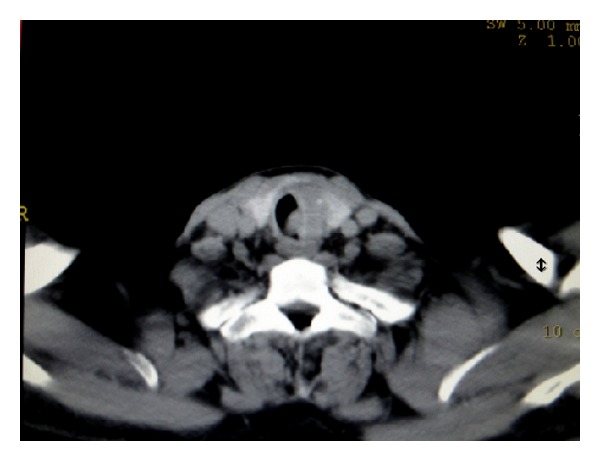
Contrast enhanced CT scan showing soft tissue mass at posterolateral wall of upper trachea (arrowhead).
